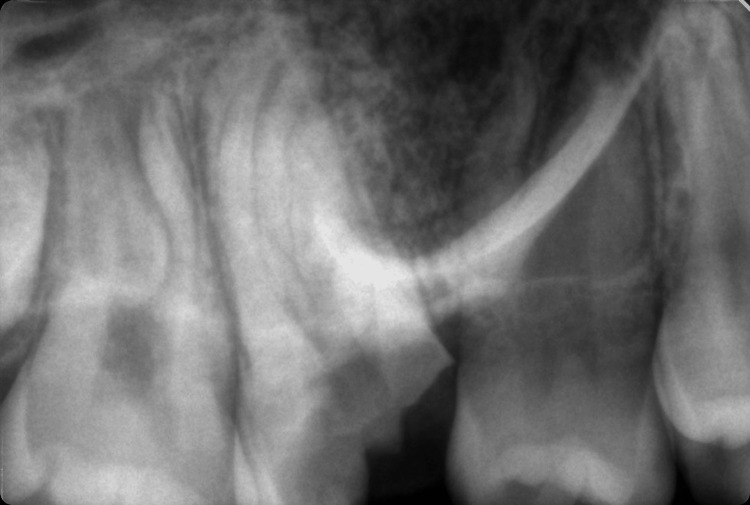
Preoperative radiographic image of tooth 16
